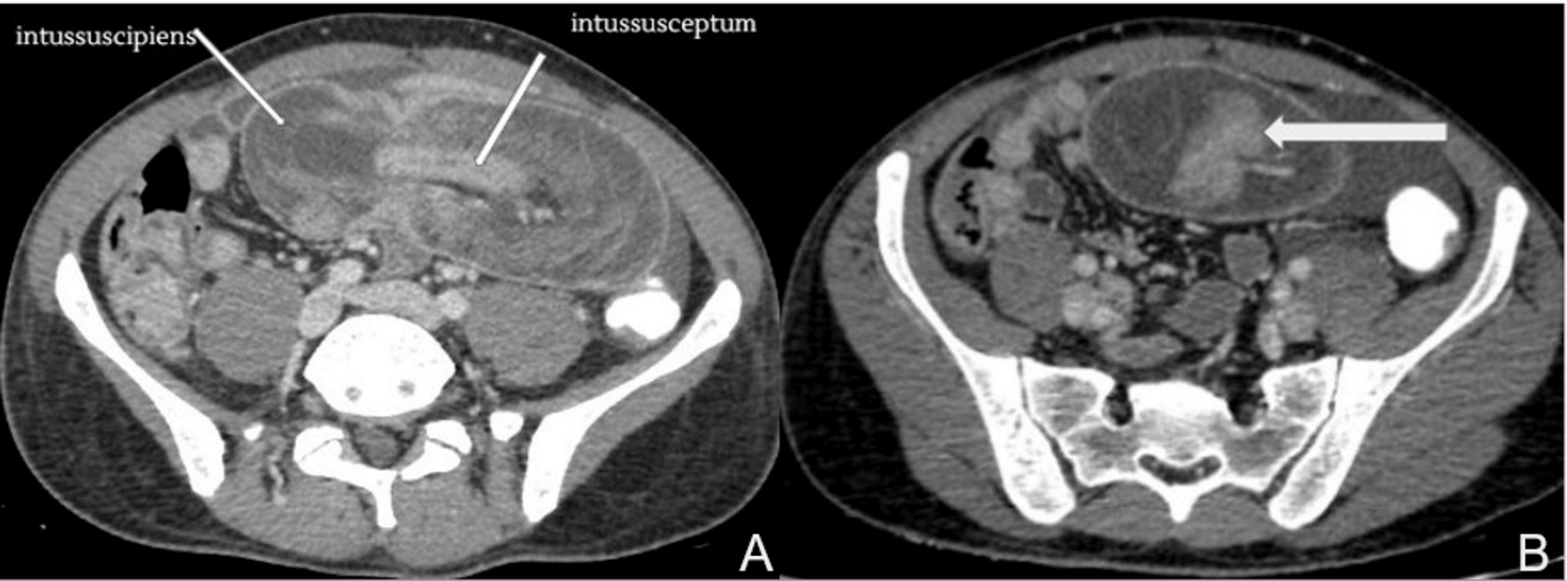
CT scan of the abdomen and pelvis with rectal and intravenous contrast on axial plane in venous phase showing (A) a bowel-within-bowel appearance involving the long segment of the small bowel (jejuno-jejunal), suggestive of intussusception with thickened and edematous wall of intussuscipiens, and (B) the largest polyp seen in the inferiormost aspect of intussusception acting as lead point (thick white arrow)
